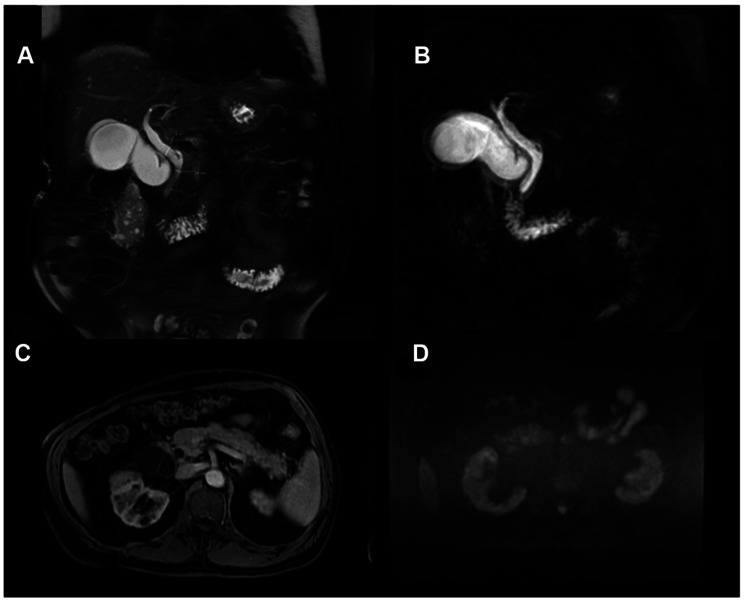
Contrast-enhanced abdominal MRI A) T2-weighted fat-saturated coronal MR image demonstrating dilation of the common hepatic duct (CHD) up to 12mm. B) 3D MRCP image confirming proximal common bile duct dilation caused by extrinsic compression from the gallbladder infundibulum. The pancreatic duct appears normal. An anatomical variant is observed, with the cystic duct inserting posteriorly and medially into the extrahepatic bile duct. C) T1-weighted contrast-enhanced image and D) diffusion-weighted imaging (DWI) sequence showing no evidence of neoplasm at the head of the pancreas.
